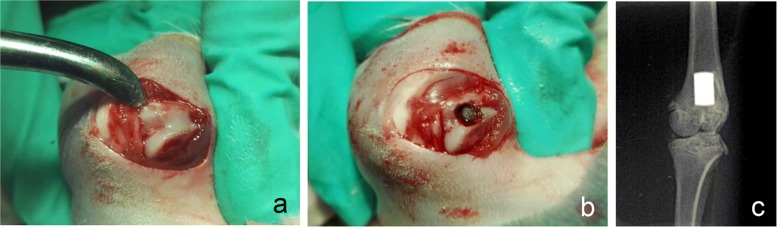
The operative location of the implant. a The exposed knee joint. b An implant was inserted into the channel. c Radiograph of the implant in the distal metaphysis of femur after surgery
